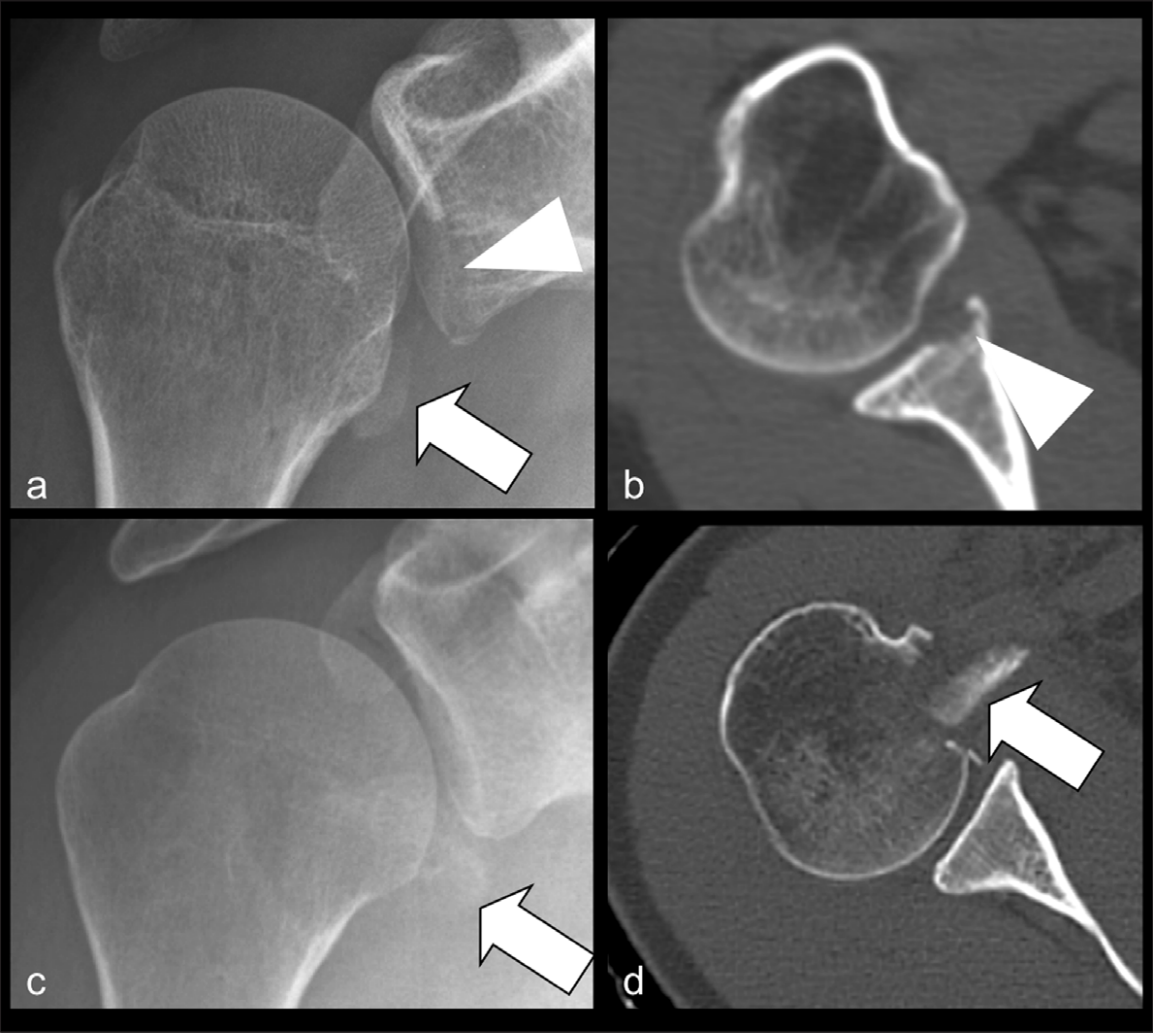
Radiographic (a) and CT (b) of bony Bankart lesion. Displaced bony fragment (arrow in a) and loss of subchondral bone plate (arrowheads). Radiographic (c) and CT (d) of fracture of lesser tubercle (arrows in c and d) mimicking a bony Bankart lesion at radiography (c).
